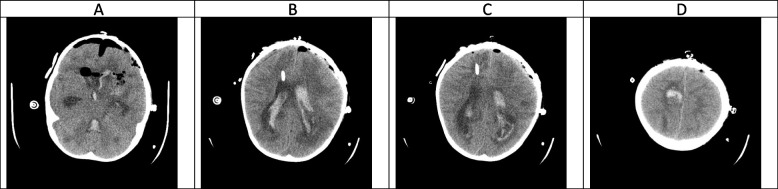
(the brain CT SCAN without contrast, day 1). A Significant air within the intracranial cavity, which is due to craniotomy. Faint hypodense lesions which were seen in the previous study are seen again in this study. Multiple air bubbles are seen at the site of the hemorrhagic lesion in the left frontotemporal lobe which is again due to the recent operation and resection of the mentioned lesion. Sign of acute hemorrhage is also seen within the third and fourth ventricles. B and C shadow of an external ventricular drain (EVD) seen traversing the right frontal lobe and entering the right lateral ventricle. Significant hemorrhage is seen within the lateral ventricles. The midline shift has been improved after the operation. D A hemorrhagic lesion is seen at the high convexity of the right frontoparietal junction without significant changes compared with the previous study
